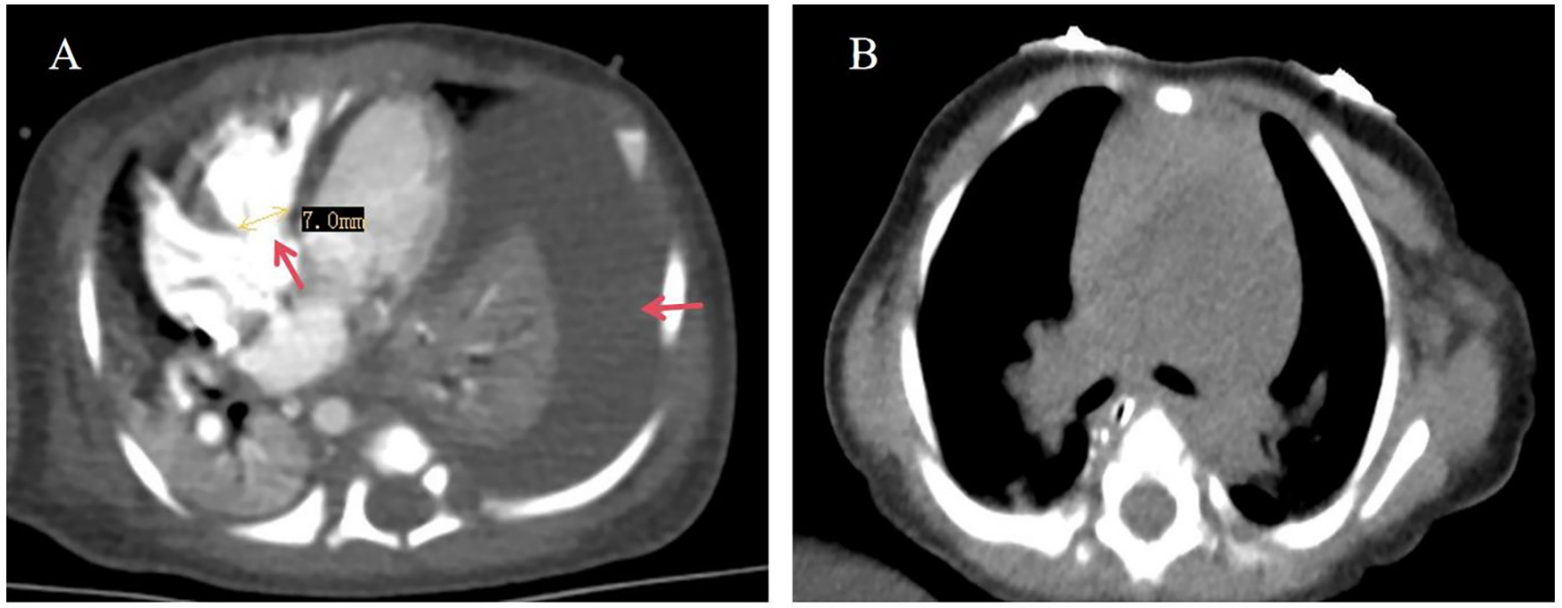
(A) Ventricular septal defect, large left pleural effusion before celiac chest sealing; (B) sealing after chest radiograph.
